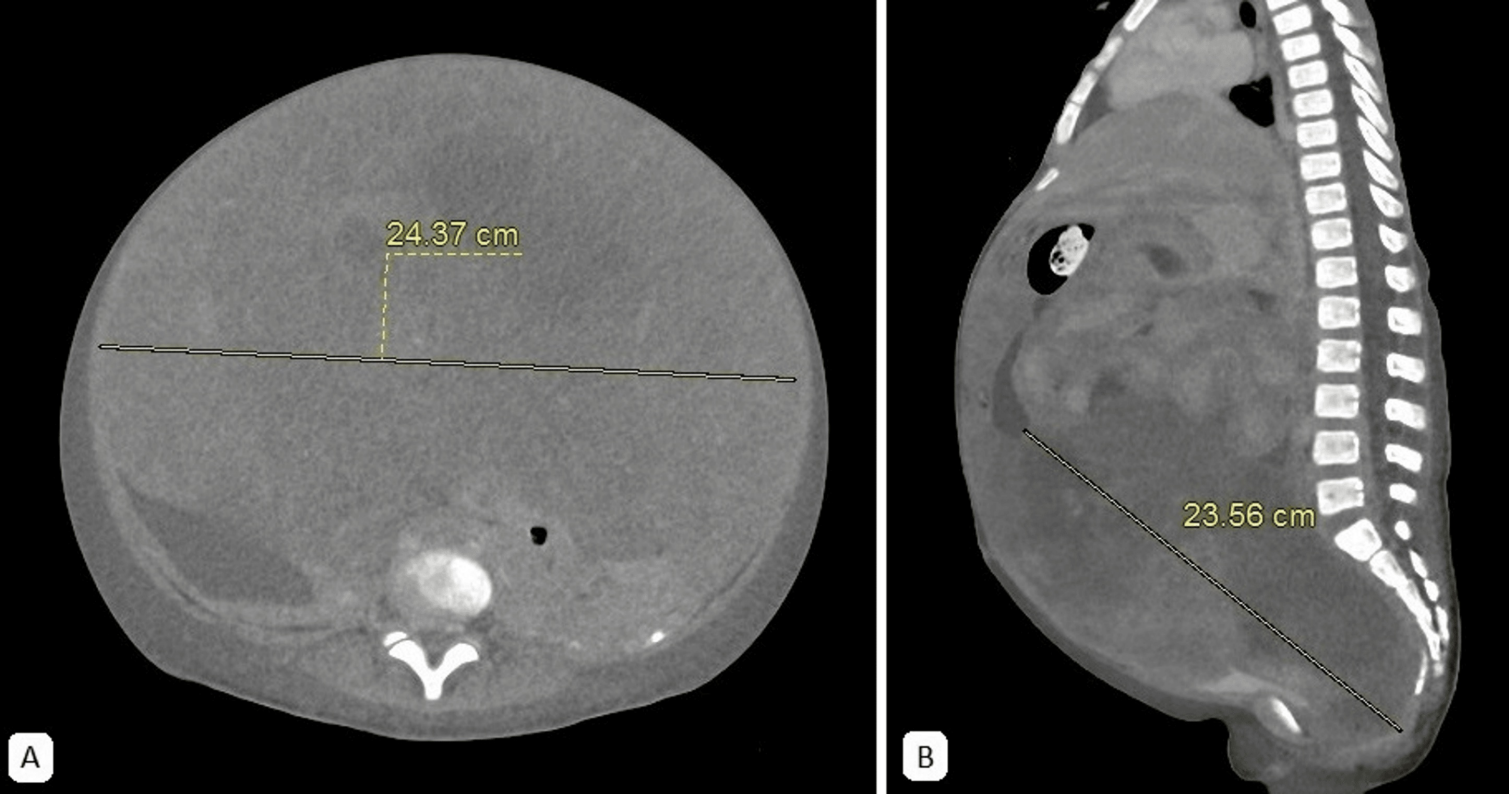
Abdominal CT scan, venous-phase. A. Axial plane. An extensive, predominantly solid mass with heterogeneous contrast enhancement is centered in the pelvic cavity and extends into the abdominal cavity. It is in close contact with the abdominal wall. B. Sagittal plane. There is diffuse thickening of the parietal peritoneum and irregular contours of the small intestine, associated with moderate free fluid in the abdominal cavity, consistent with peritoneal extension.
